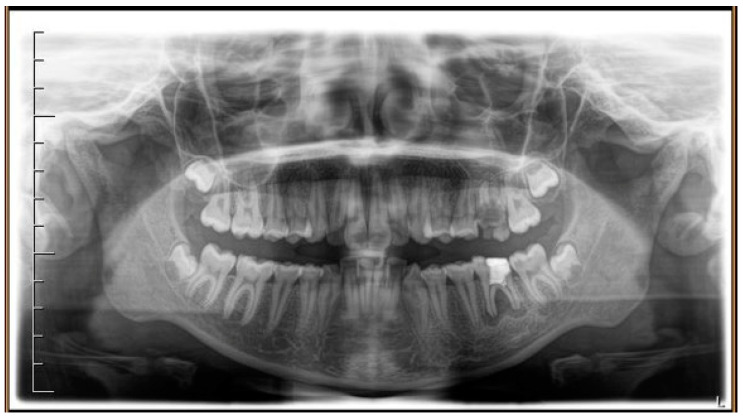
Pre-treatment radiograph of the patient. The first two left molars appear irreparably compromised by destructive caries.
